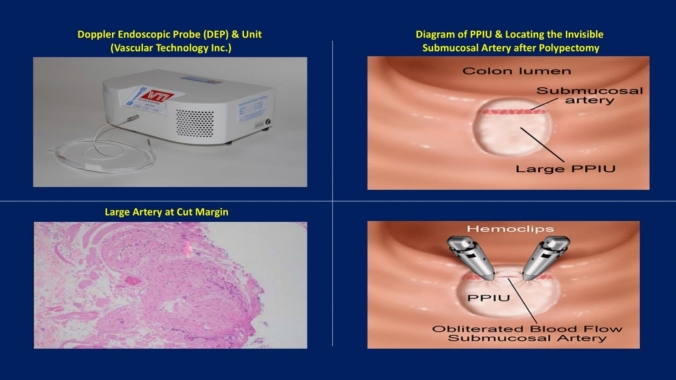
Results of interrogating the base of a post-polypectomy ulcer with Doppler endoscopic probe to detect arterial blood flow and map a large invisible submucosal artery
